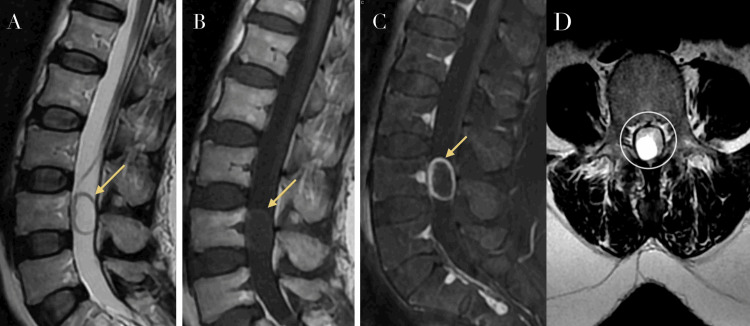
Lumbar spine MRI showing a cystic intradural lesion (A) T2-weighted image showing a hyperintense intradural lesion anterior to L4 (yellow arrow). (B) T1-weighted image demonstrating corresponding hypointensity (yellow arrow). (C) Post-gadolinium T1 image with peripheral rim enhancement (yellow arrow). (D) Axial T2 MRI showing a hyperintense intradural lesion with mild mass effect, consistent with a cystic tumor (yellow arrow).
